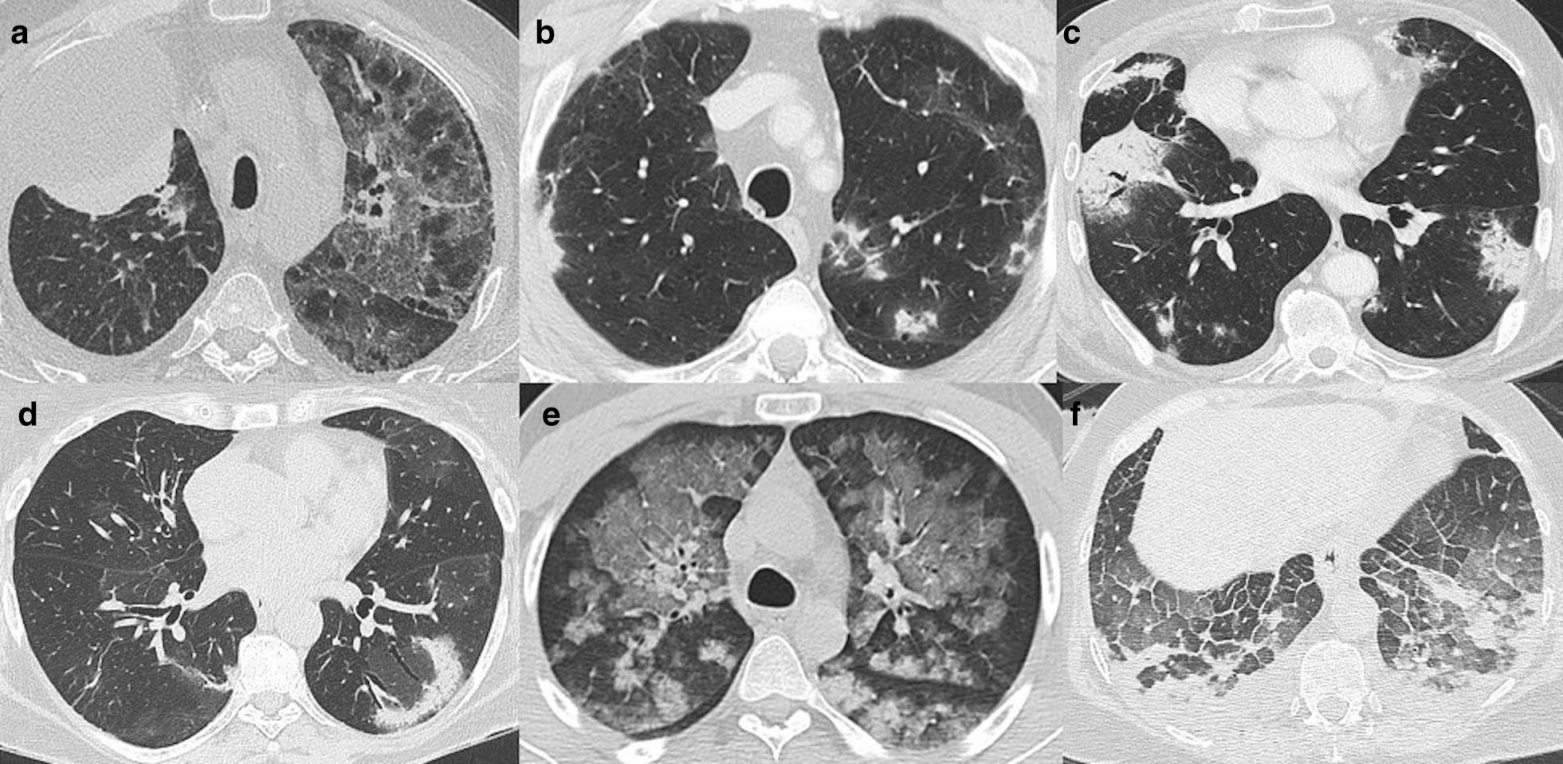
Axial CT images of different cases of ChT/RT-related lung toxicity. a shows acute pneumonitis after the first administration of an anti-PD-1 target immunotherapic agent (Pembrolizumab) in a patient with right lung cancer. Also Nivolumab (another anti-PD-1 agent) can cause lung adverse disease with interstitial pneumonitis presenting with organizing pneumonia pattern, as shown in (b, c). An example of radiation-primed cryptogenic organizing pneumonia is reported in (d), which shows the so-called “reverse halo sign” or “atoll sign” in the left lower lobe after RT for breast cancer. e Shows acute and diffuse alveolar hemorrhage in a patient with NSCLC treated with Bevacizumab, with bilateral GGOs caused by blood filling alveolar spaces. Acute lung edema with bilateral smooth lobular septal thickening and GGOs in a patient treated with IL-2 is shown in (f)
